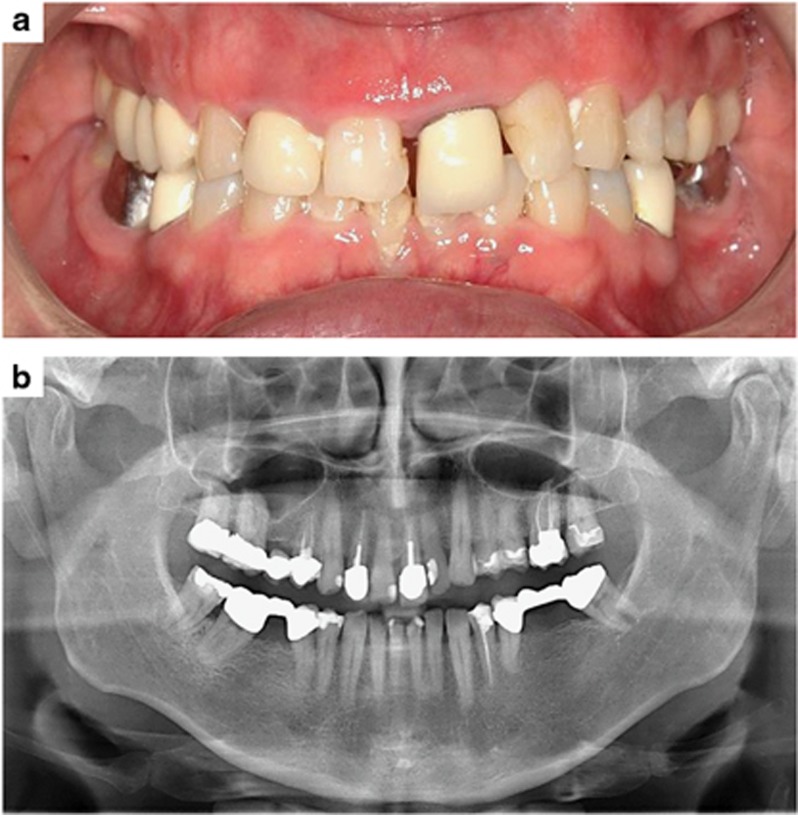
Adult orthodontic patient with a long-term consumption of more than 10 cigarettes per day, requiring an orthodontic correction of the dental deep bite and misaligned first upper incisor. (a) Intraoral view; (b) panoramic radiograph of the dentition and alveolar jaw bone. The gingival tissue shows signs of inflammation and a general horizontal periodontal bone loss due to a chronic nicotine-induced periodontitis is evident.
